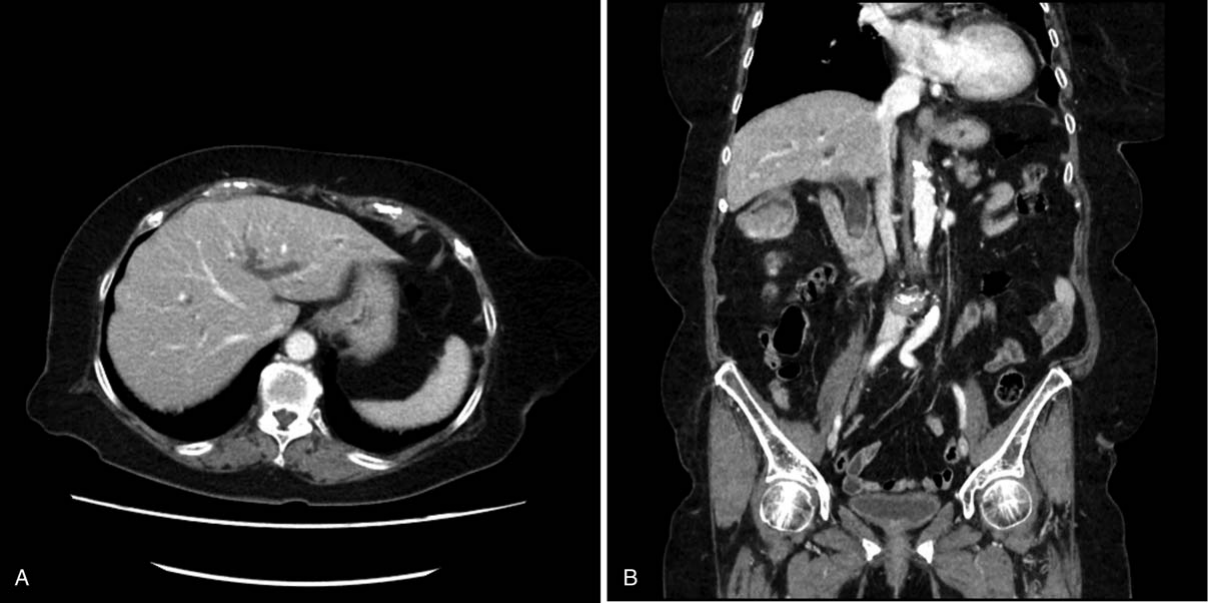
Computed tomography showing dilatation of intrahepatic duct (A) and common bile duct stone (B).
